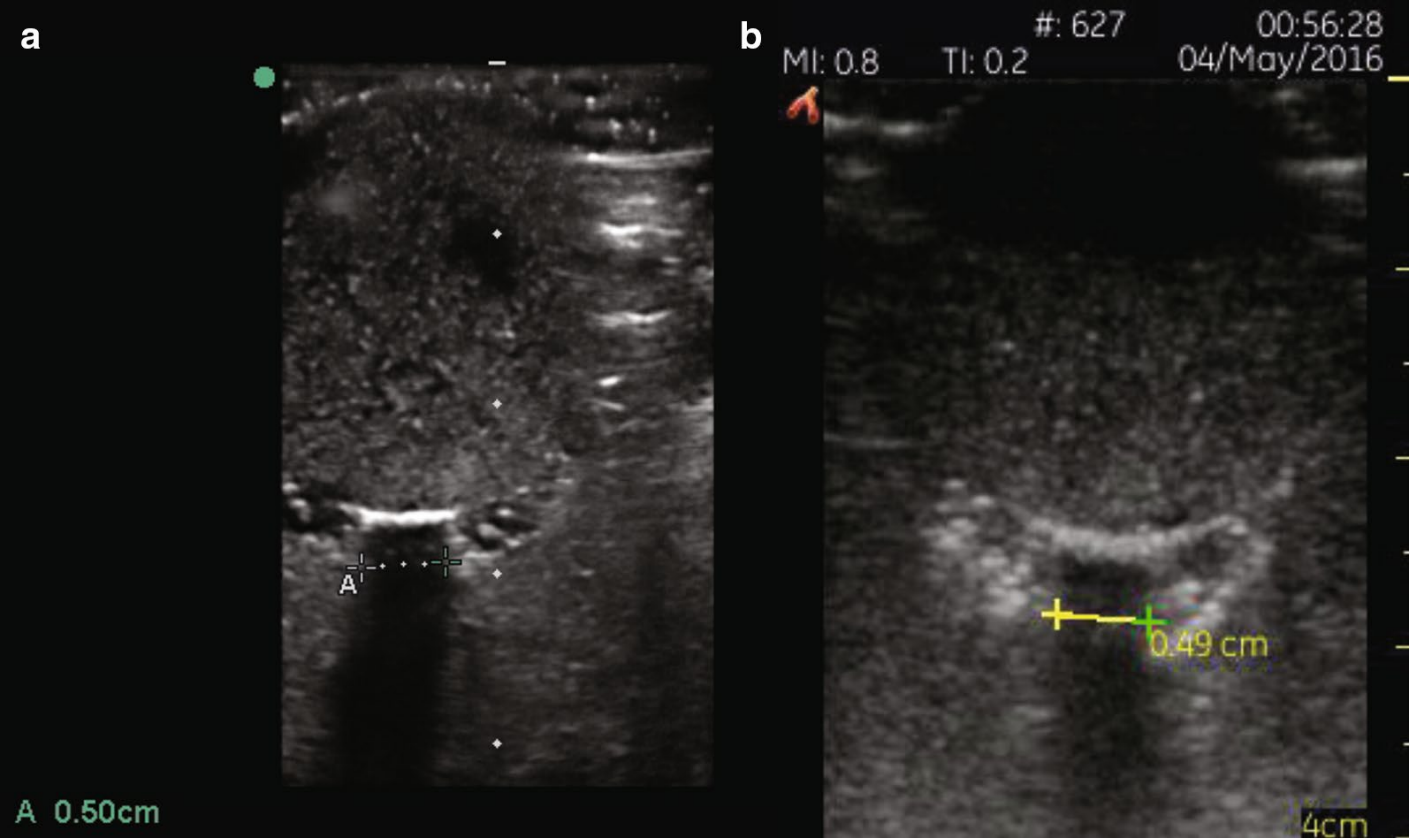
Ultrasound images of ONSD measurements utilizing the SonoSite (a) and Vscan (b) ultrasound units
